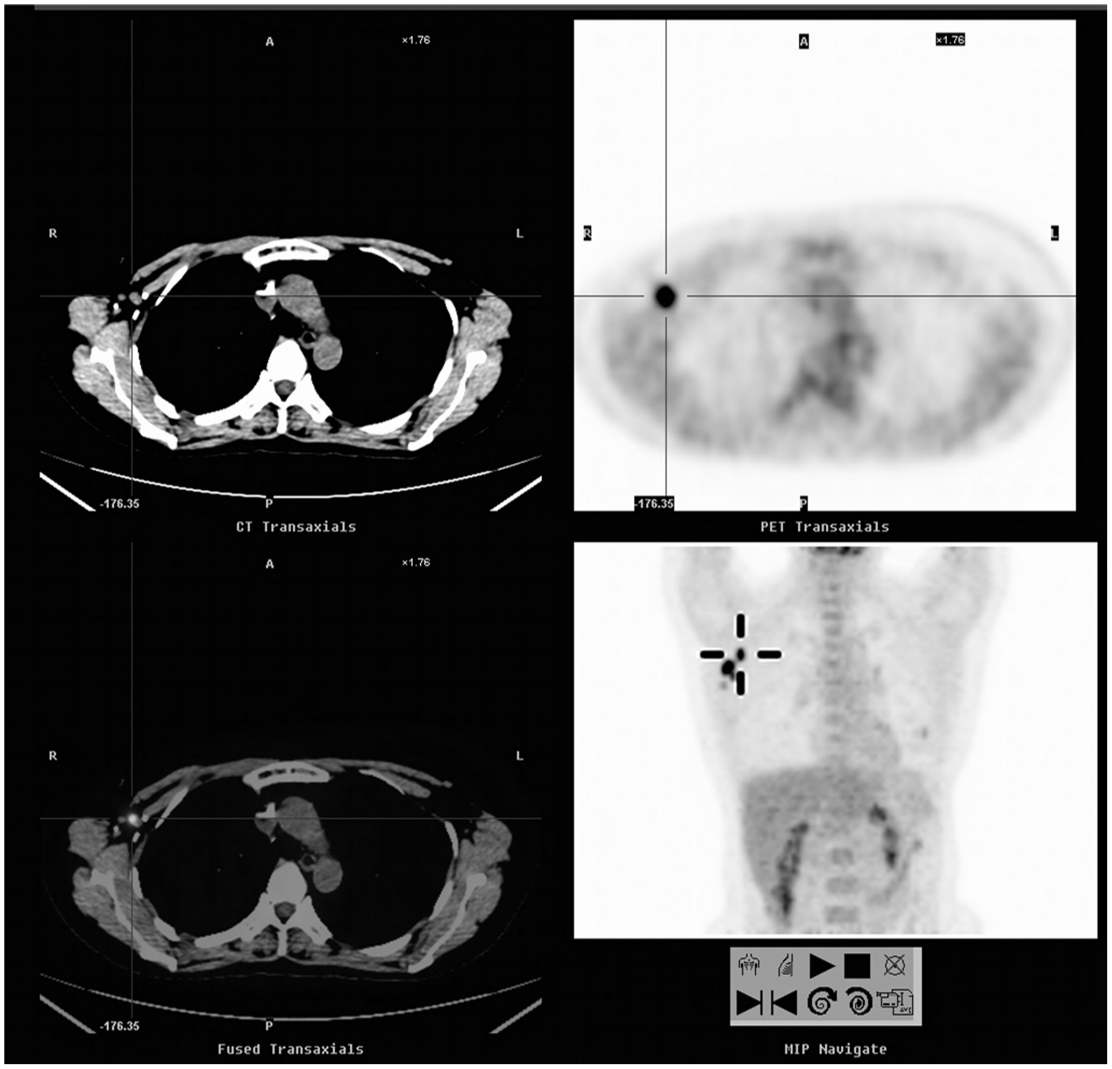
A 46-year-old woman with a history of breast cancer (invasive ductal carcinoma of the right breast, stage IIIA) underwent a FDG-PET/CT scan for asymptomatic follow-up. FDG-PET/CT showed glucose hypermetabolism in the right axillary nodes (SUVmax = 7.7, cross cursor). The pathological analysis confirmed the presence of metastatic lymph nodes. The patient's serum CA 15-3 remained within normal ranges throughout the course. The patient remained on clinical follow-up at the end of the study.
